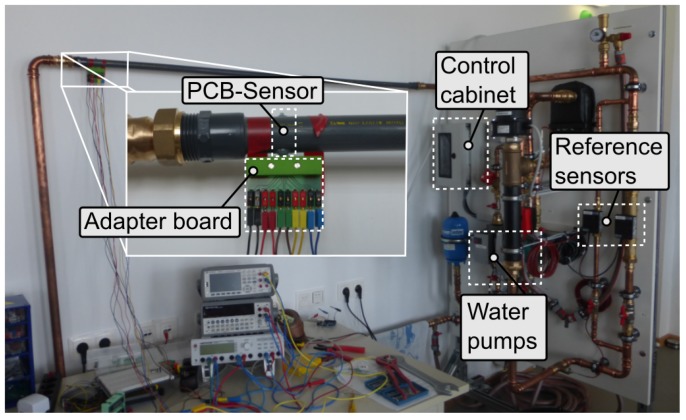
Picture of the water experimental setup. The sensor is stretched across a PVC water pipe (inner diameter of 21.2 mm), located at 135 cm at a strait pipe which is 20 cm before a bend, and connected to the controller by an adapter board. The volumetric flow is controlled via two separate water pumps and measured with two ultrasonic flow sensors. From a control cabinet, all sensors and actuators are controlled.
